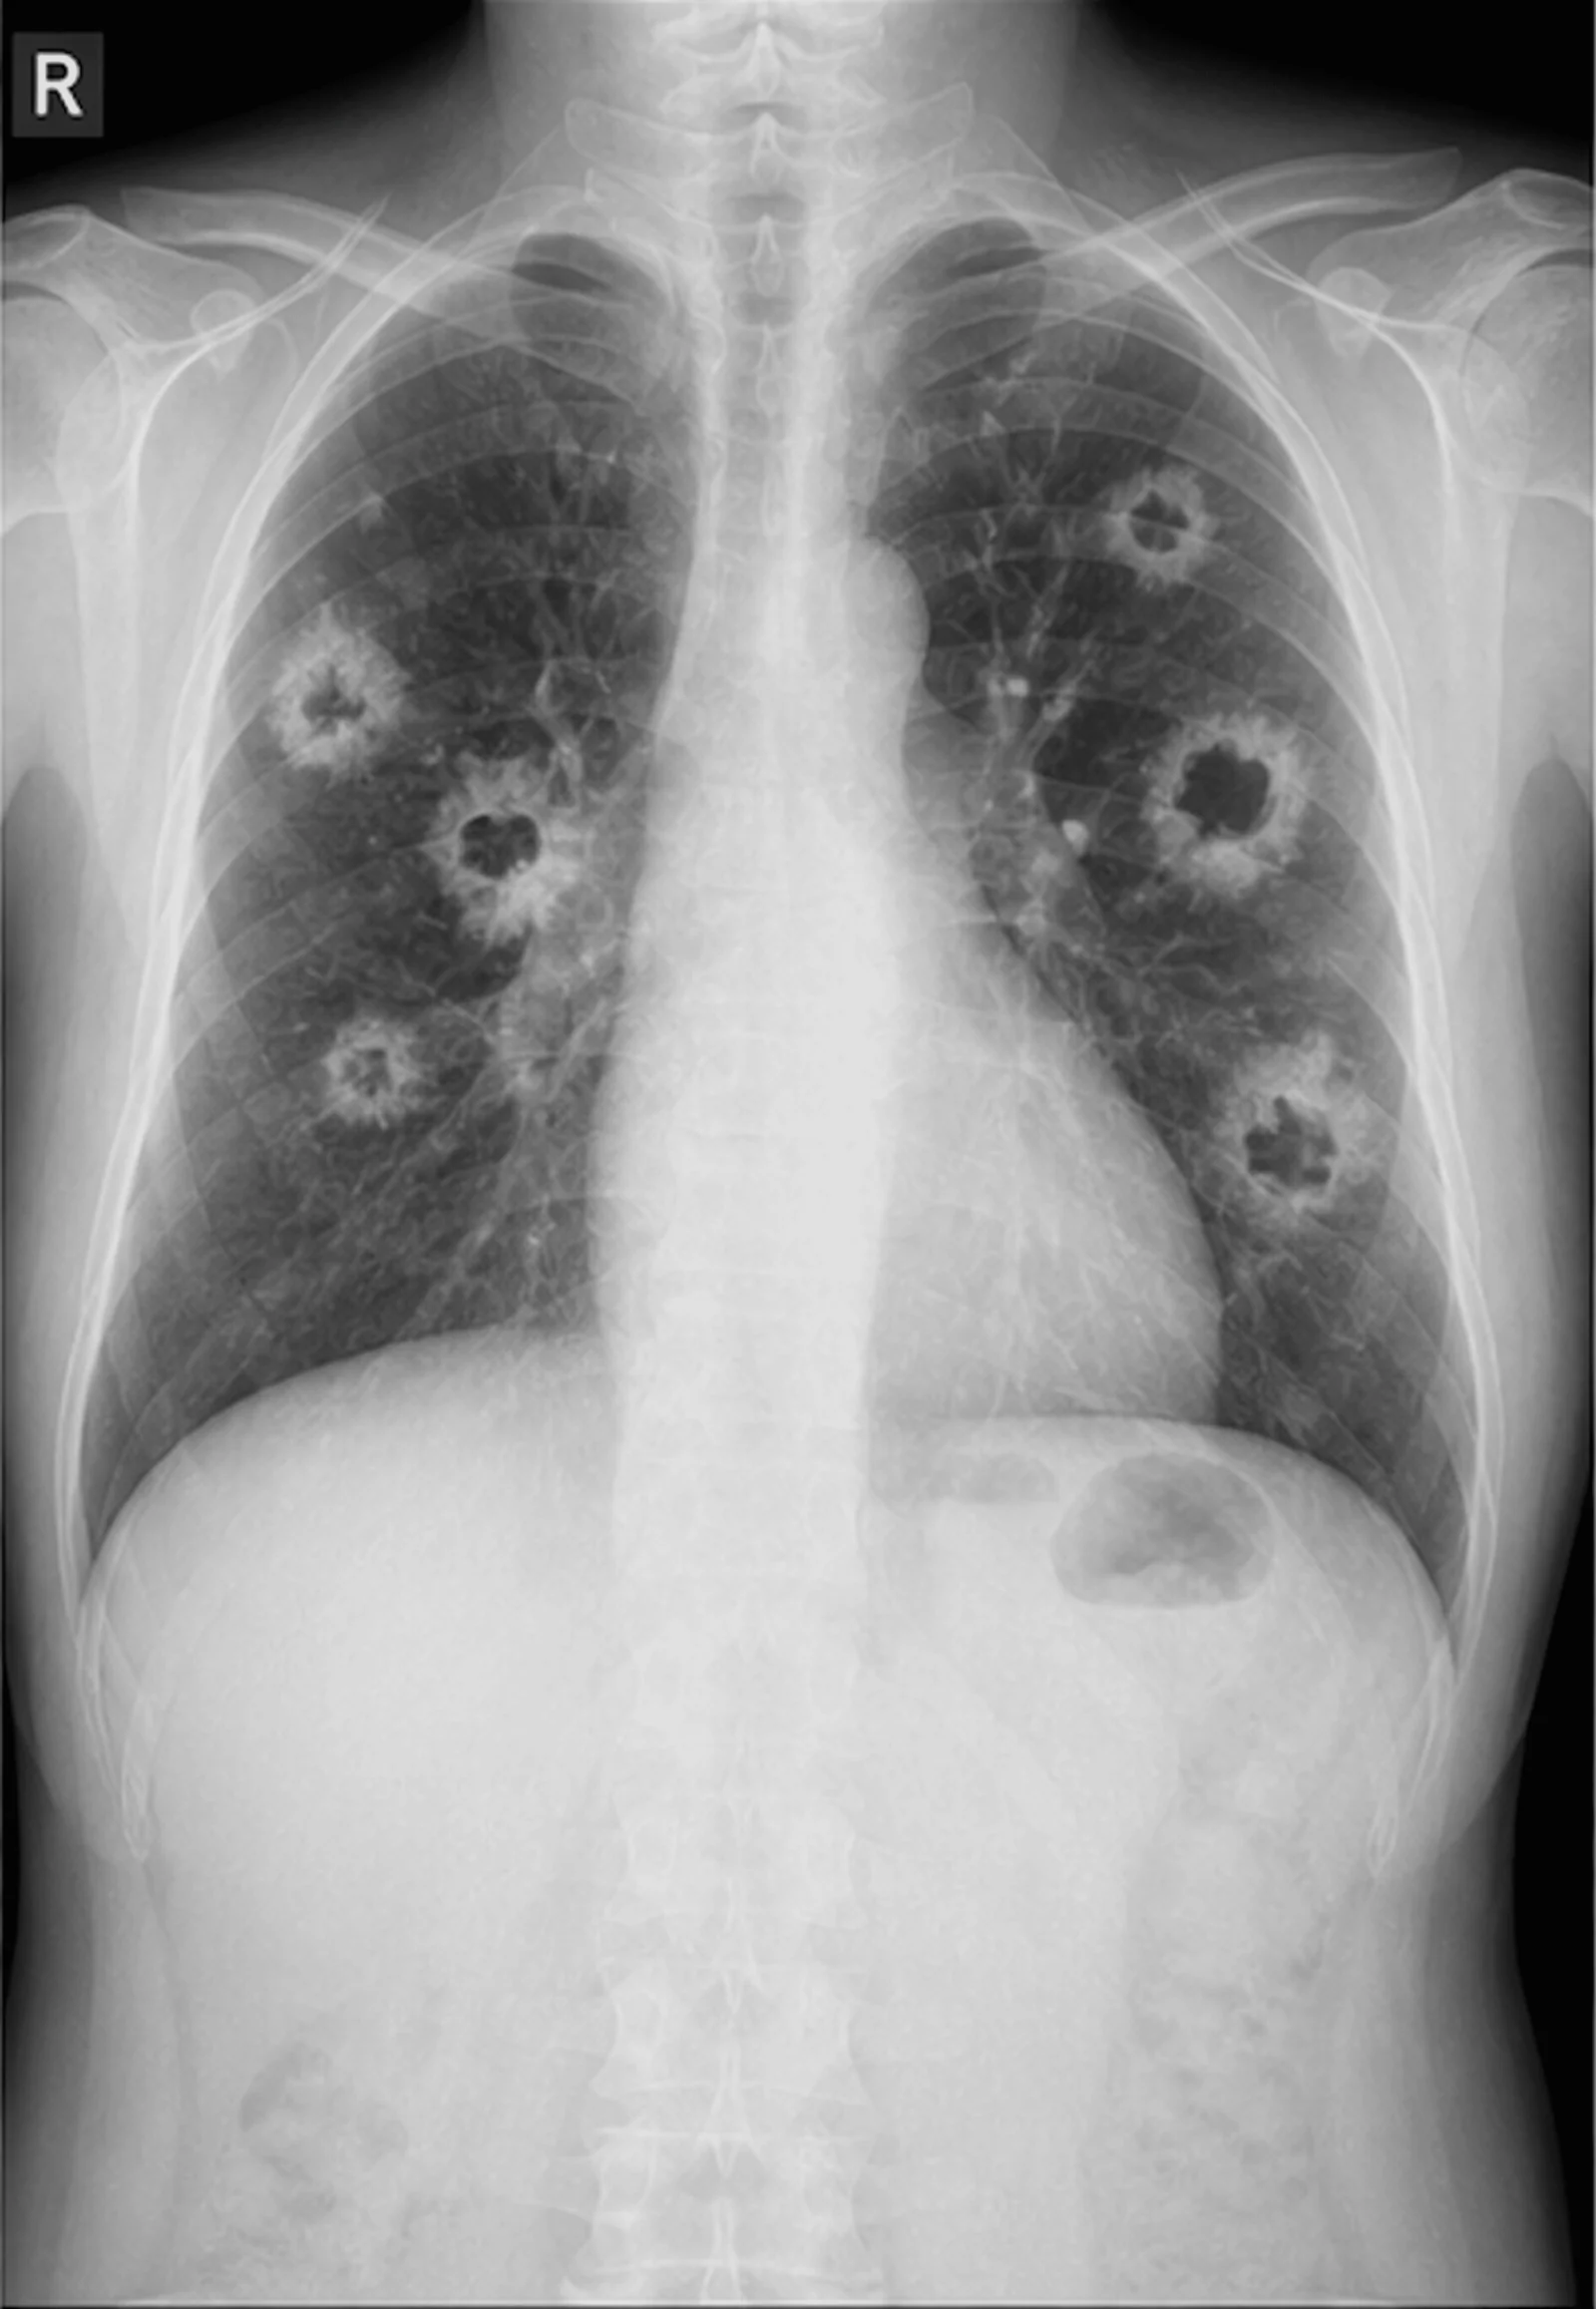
Chest X-ray Chest X-ray revealed multiple bilateral nodular opacities with cavitations.
